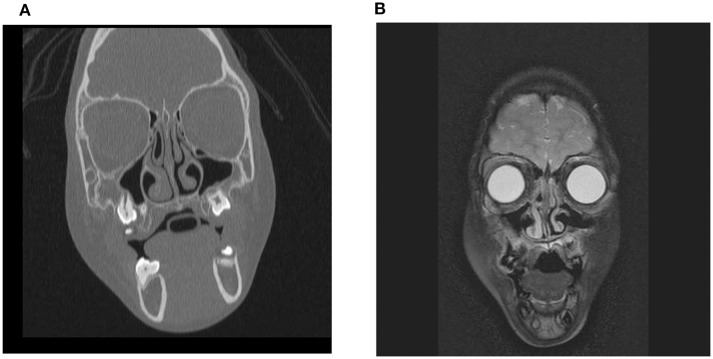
Facial MRI (A) and CT (B) scans performed in the patient with WS when she was 5 years old. No significant asymmetry between the maxillary sinuses was observed.
